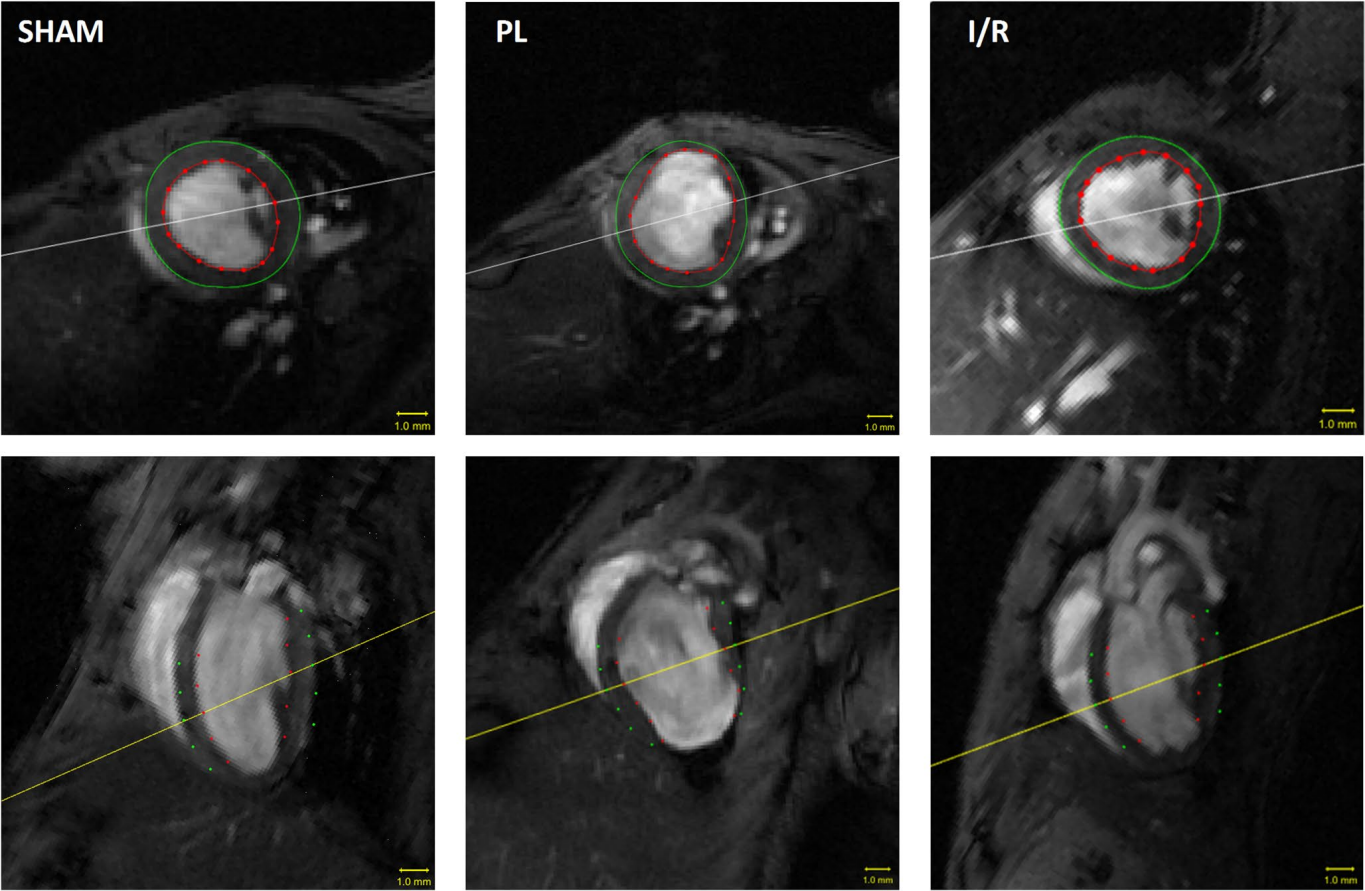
Representative end-diastolic (ED) MRI images of the left ventricle 4 weeks after SHAM, PL or I/R surgery. Top row: short axis view. Bottom row: long axis view. The epicardium is marked with green dots, the endocardium with red dots. Scale bar: 1 mm
